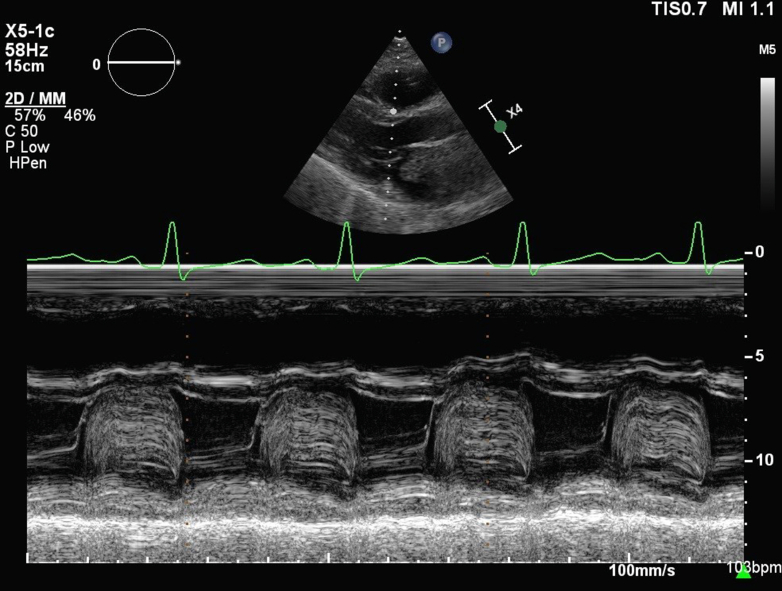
Transthoracic M-Mode Tracing of the Mitral Valve
